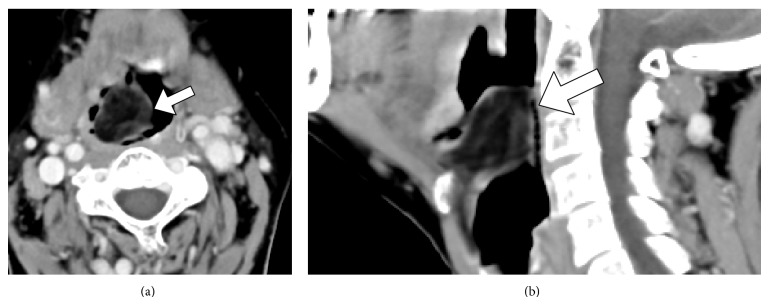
Contrast-enhanced (a) axial and (b) sagittal CT images showed a lipoma occurring on the lingual surface of the epiglottis (arrows).
